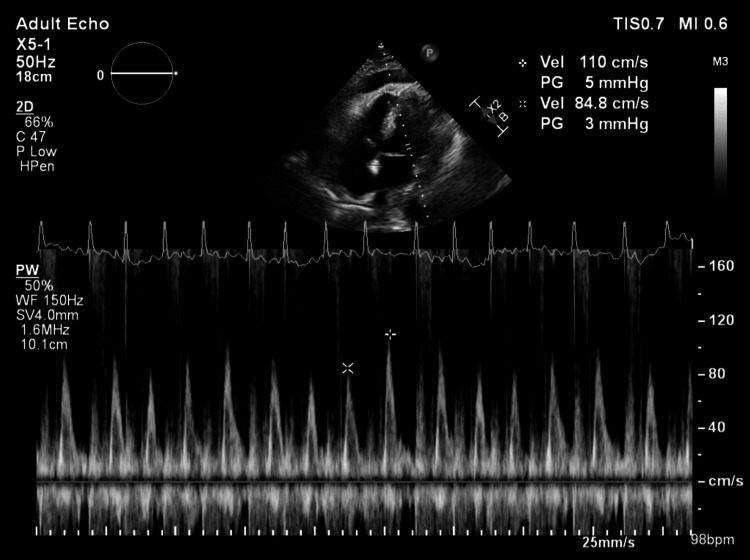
Transthoracic echocardiography with pulsed-wave Doppler across mitral inflow in apical four-chamber view demonstrating increased mitral transvalvular velocity variation during respiration, suggestive of cardiac tamponade The marked arrows show peak velocity of 110 cm/s (5 mmHg gradient) during inspiration and 84.8 cm/s (3 mmHg gradient) during expiration, showing increased respiratory variation in mitral inflow velocities, which is suggestive of tamponade physiology.
